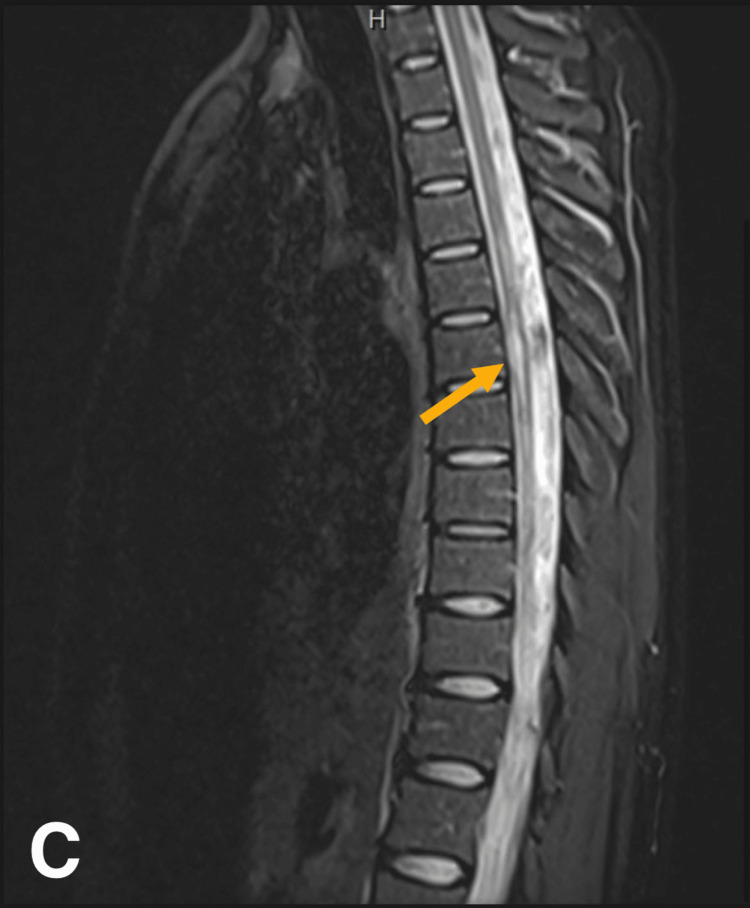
Spinal MRI showing short segmental enhancing lesion at T6-T8 (arrow) correlating with the T7 sensory level
